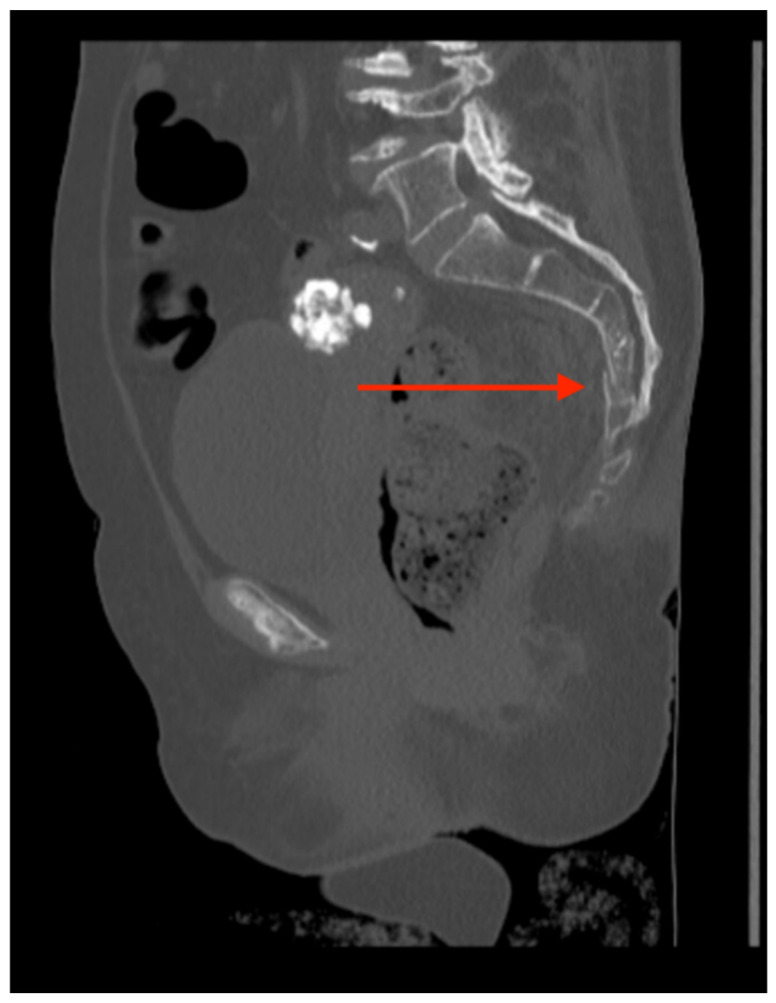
CT abdomen/pelvis images of the described fracture in case 5. Red arrows are used to indicate the location of pelvic fractures for easier identification.
